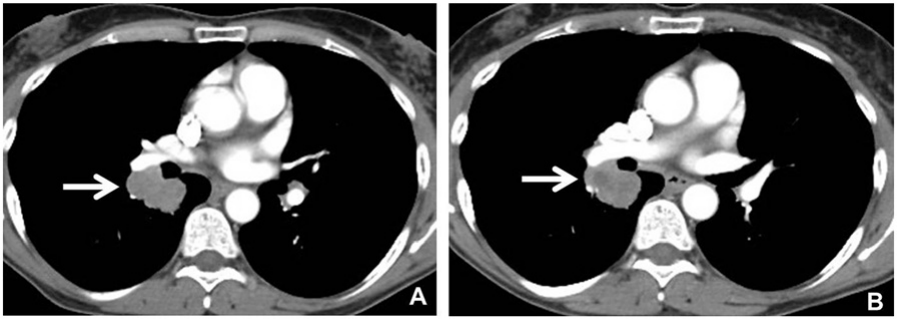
A 61-year-old male with metastatic melanoma. Contrast-enhanced CT scan of the chest at baseline (A) demonstrate a lobulated right lower lobe mass measuring 37 mm and 54.3 HU (A, arrow). The follow-up scan (B) at 11.4 weeks of ipilimumab and bevacizumab therapy demonstrated the lesion measuring 36 mm and 44.4 HU (B, arrow). While the percent decrease of diameter was only 2%, CT density decreased by 18% comparing to baseline, meeting the Choi response criteria.
